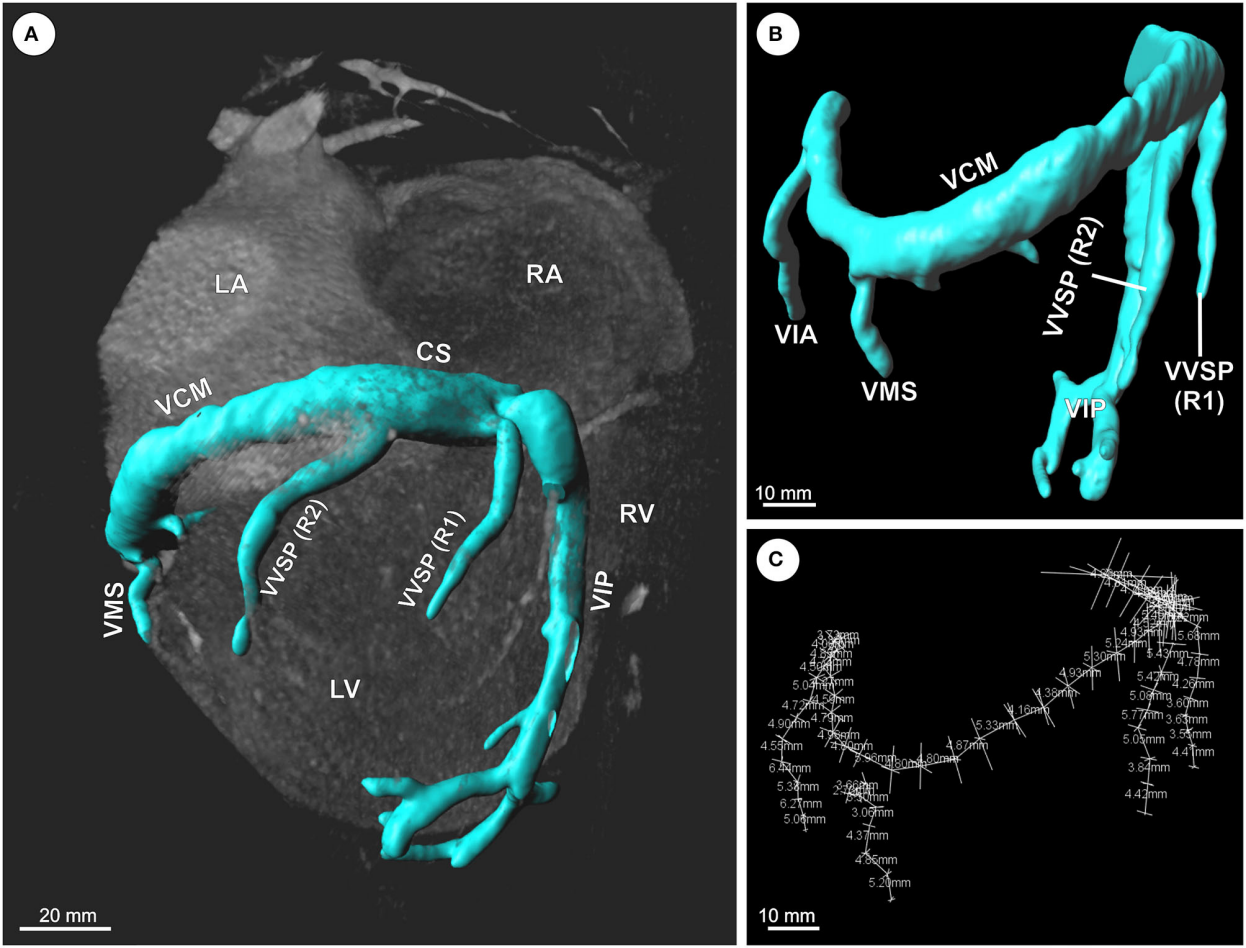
(A–C) Exemplary 3D reconstruction and morphometric analysis of the coronary vein system (CVS) based on CT datasets. (A) Volume rendered CT image of the heart with overlaid surface model of the CVS. (B) Reconstructed surface model of the CVS with overlaid orthogonal measurements every 5 mm. (C) Measurement points and lines along the CVS. CS, coronary sinus; LA, left atrium; LV, left ventricle; RA, right atrium; R1/R2, 1st/2nd ramus; RV, right ventricle; VCM, great cardiac vein; VIA, anterior interventricular vein; VIP, posterior interventricular vein (=middle cardiac vein); VMS, left marginal vein; VVSP, left posterior ventricular vein.
